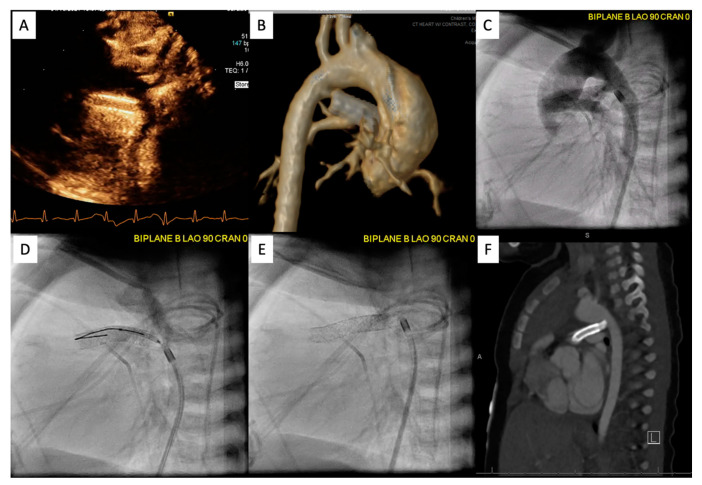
Patient is status post PDA stenting with concern for cyanosis. ECHO/CT scan demonstrated that the aortic end was uncovered (A,B). Patient returned to the catheterization lab for an additional stent placement (C–E). Follow up PDA stenting showed full coverage (F).
